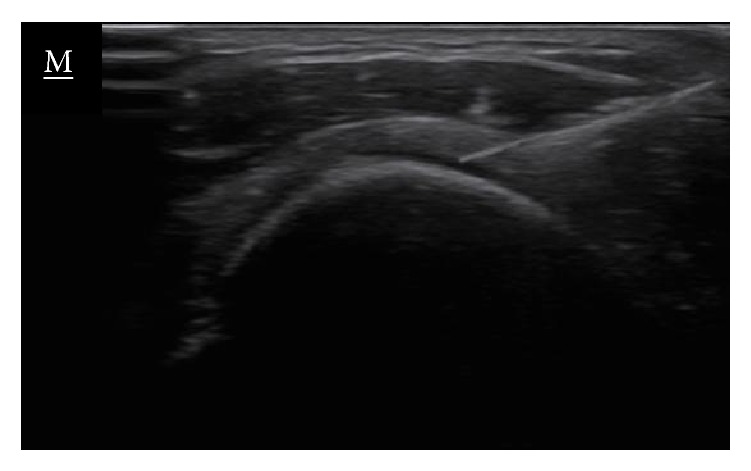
Long-axis grey-scale ultrasound image displaying the supraspinatus tendon during needle placement showing the echogenic needle during the application of US-guided percutaneous electrolysis.
